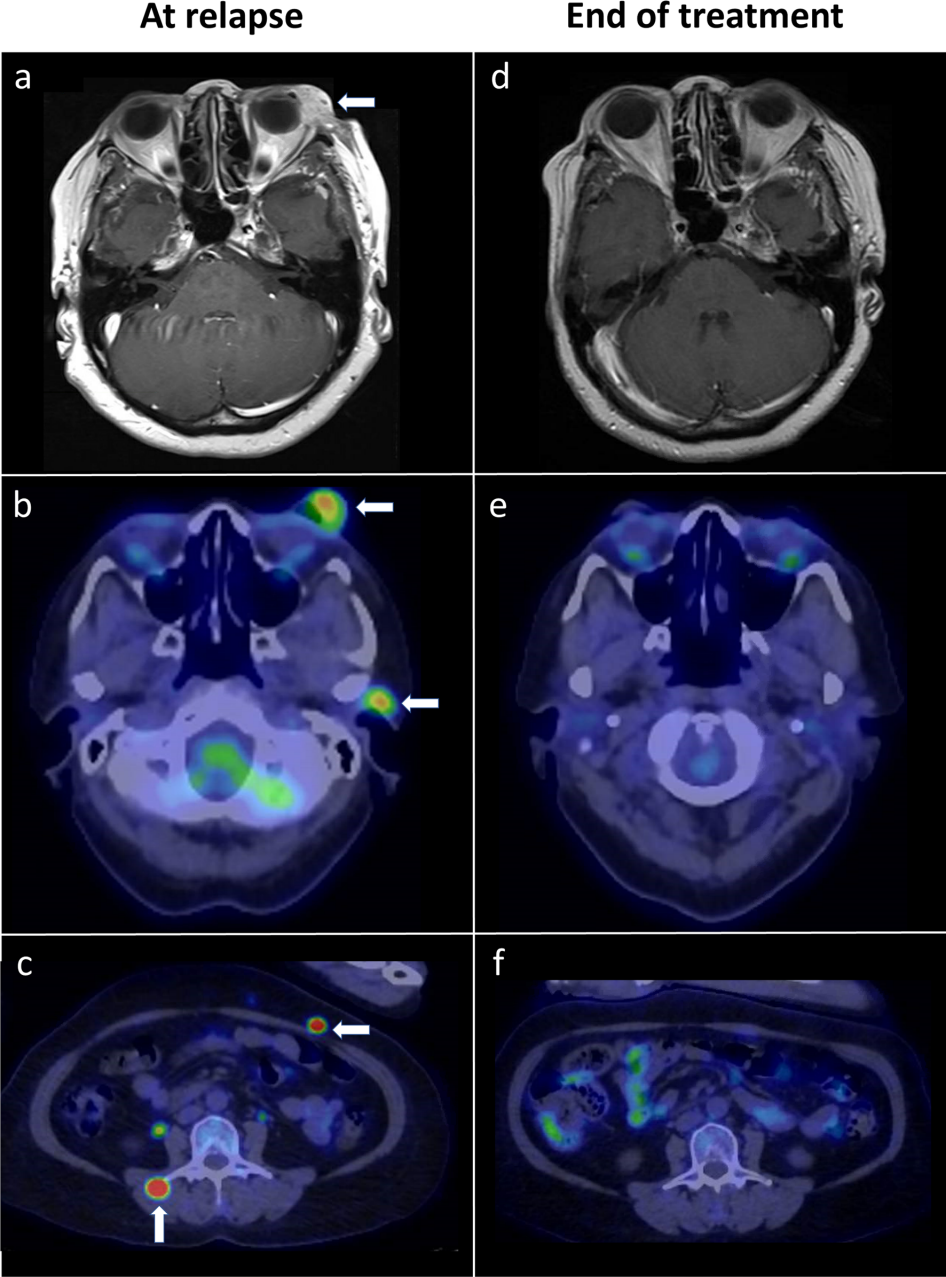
Complete response to Pembrolizumab in recurrent metastatic conjunctival melanoma. (a) MRI brain showing a large enhancing mass arising from the left conjunctiva enveloping the left lacrimal gland, at the point of relapse before treatment. (b) Corresponding PET-CT images demonstrating hypermetabolic tumours of the left conjunctiva, left parotid lymph node, (c) abdominal wall as well as erector spinae muscles. (d–f) Complete radiological and metabolic response was achieved after 2 years of Pembrolizumab.
